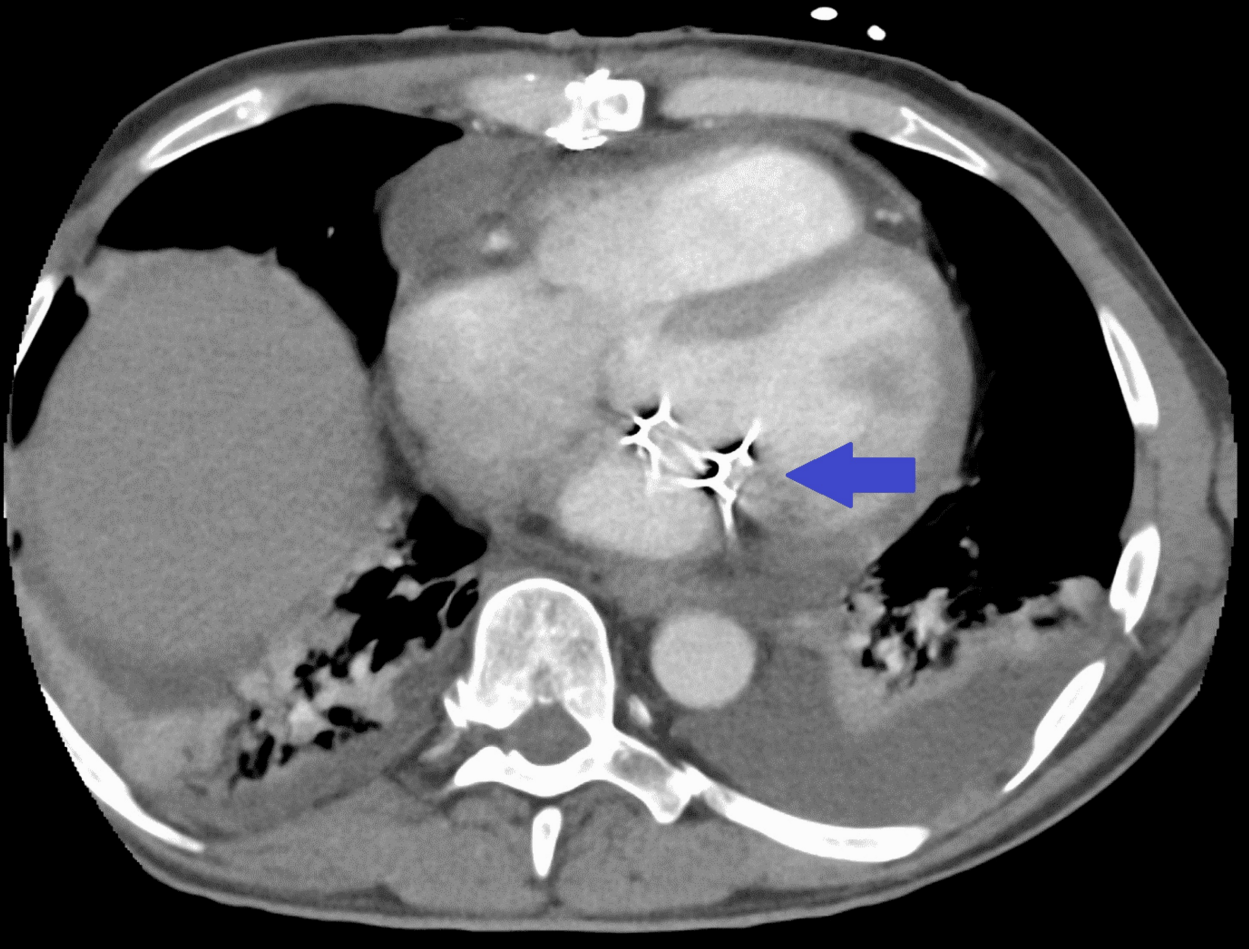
CT scan showing mitral valve replacement with mechanical prosthesis (blue arrow).
